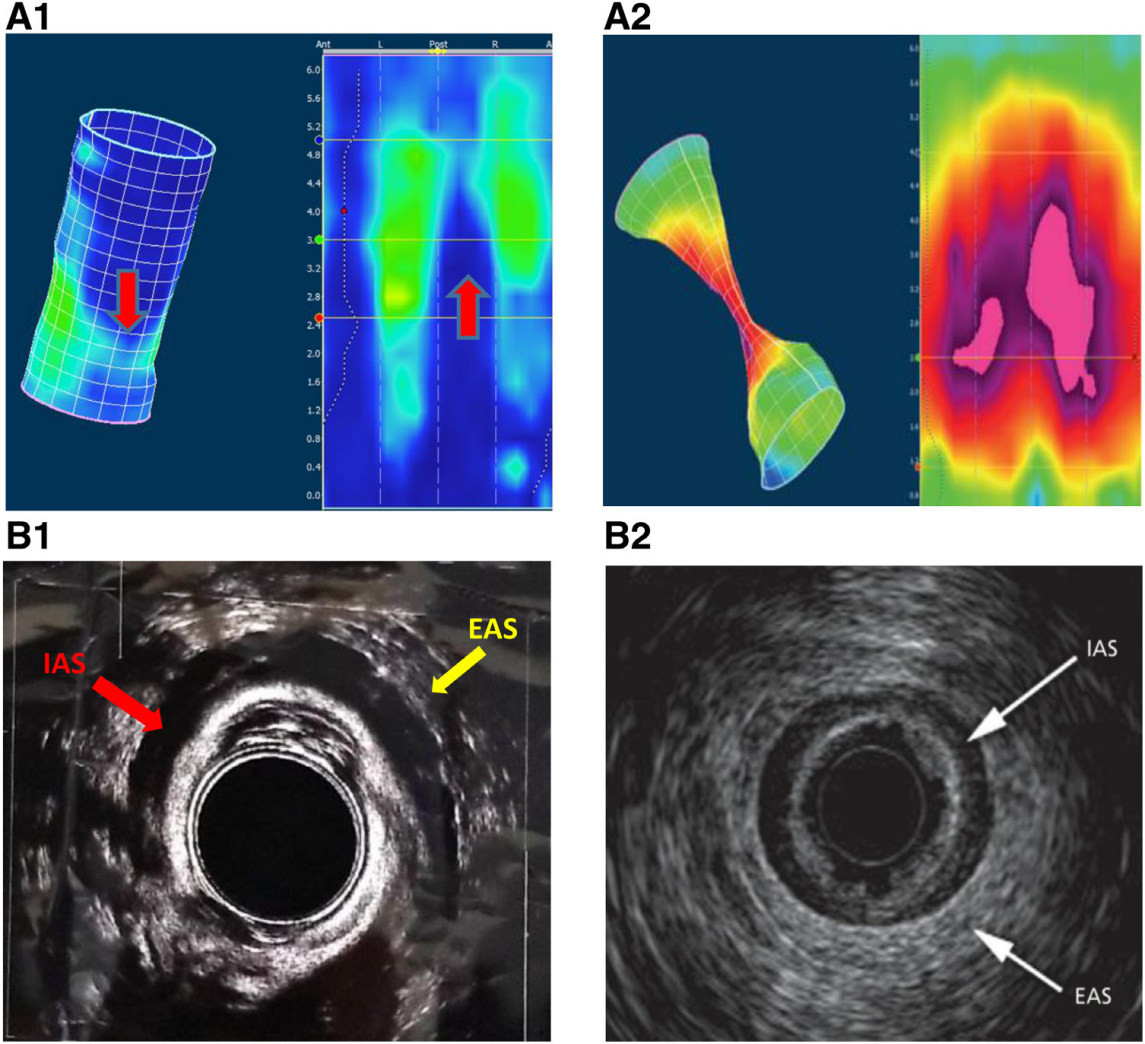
(A1) Shows 2D and 3D images from HRAM showing sphincter defects indicated by low pressures (red arrows). (A2) shows a normal 2D and 3D anorectal manometry5. (B1) TRUS shows both the intact parts of the IAS, (red arrow—inner hypoechoic ring) with defect extending from 3 to 9 o’clock and EAS, (yellow arrow—outer hyperechoic ring) with a defect from 5 to 8 o’clock. (B2) shows a normal TRUS image of normal sphincters. Normally the IAS and EAS are complete circles with no interruption. EAS = external anal sphincter; HRAM = high resolution anorectal manometry; IAS = internal anal sphincter; TRUS = transrectal ultrasonography.
